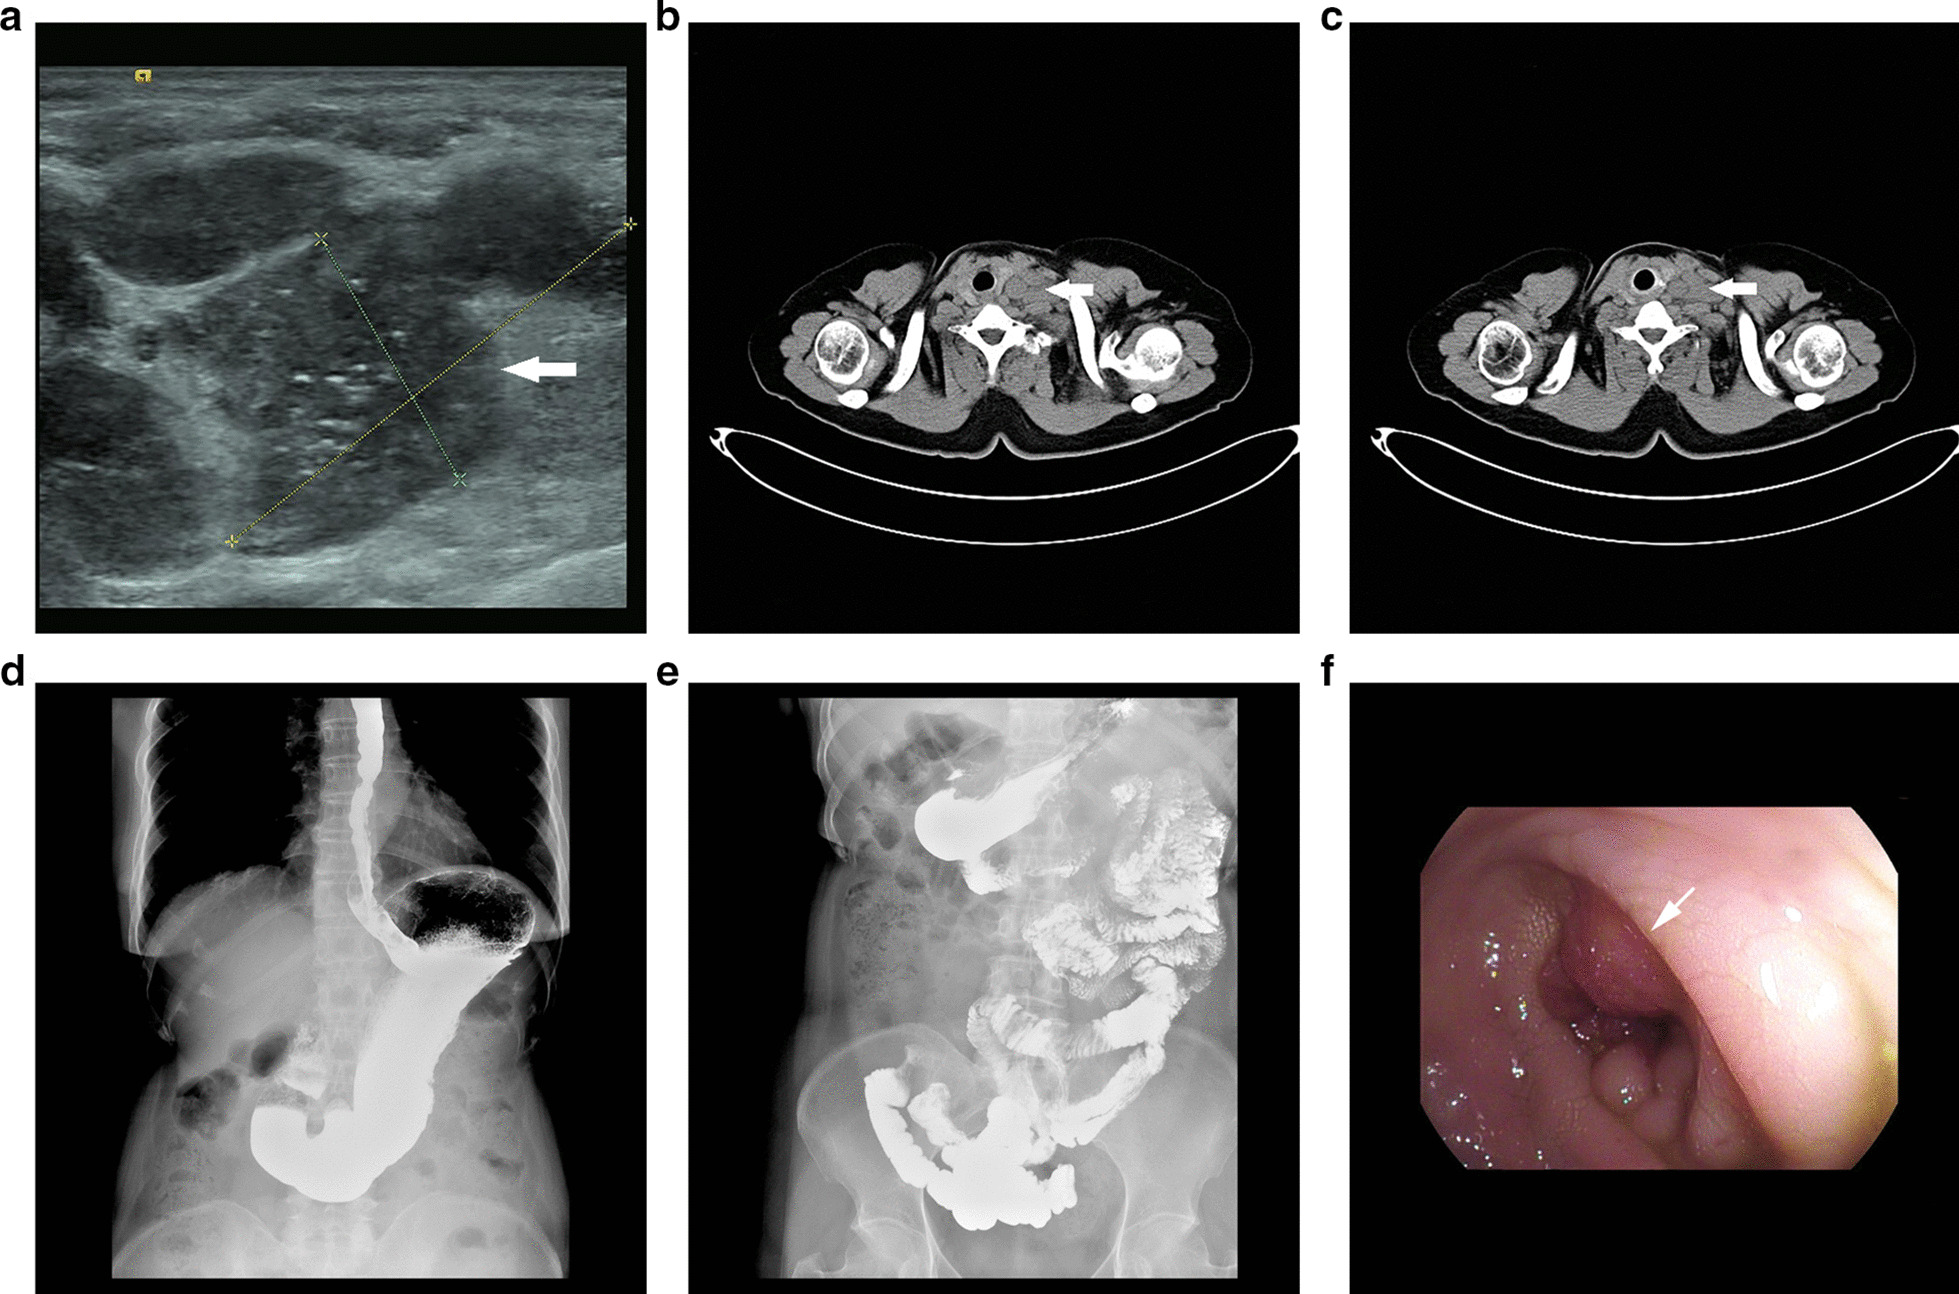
a (Cervical ultrasound) showed multiple hypoechoic nodules in the left neck and left supraclavicular fossa with clear boundaries, irregular morphology, multiple dotted strong echoes and rich blood flow signals. b, c (Chest computed tomography) showed multiple enlarged lymph nodes in the bilateral submandibular region, bilateral carotid sheath and intermuscular space of the cervical root. d (Whole gastrointestinal tract barium X-ray radiography) showed whole gastrointestinal tract has no sign of obstruction. e, f (Lower gastrointestinal endoscopy) showed irregularly elevated rectal mucosa with congestion, erosion and easy bleeding. The lesion caused rectal narrowing and the endoscopy was not able to pass through
